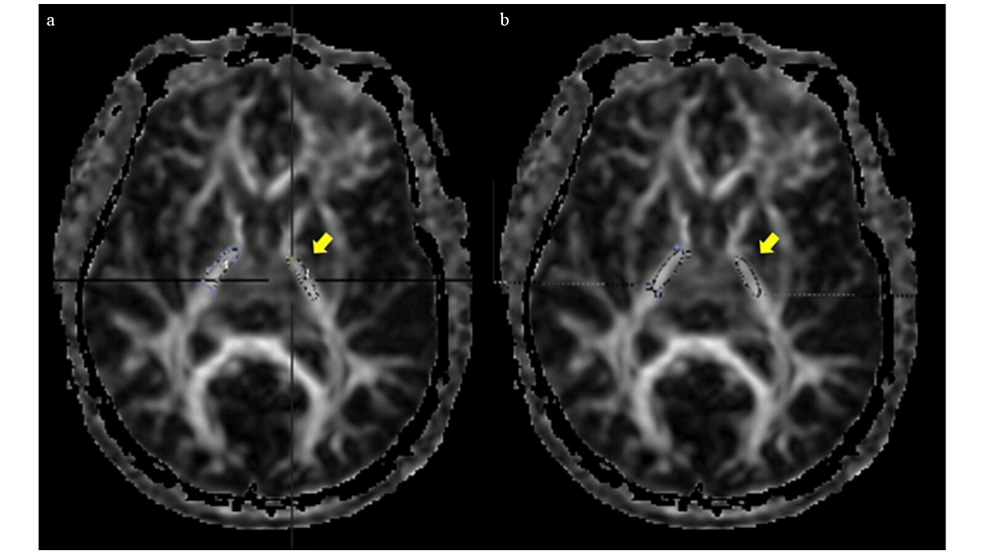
Example of how ROI is measured. (a) ROI of the internal capsule on DTI, (b) ROI of the internal capsule on synthesized DTI and SPECT. ROI: regions of interest, DTI: diffusion tensor image, SPECT: single photon emission computed tomography.
